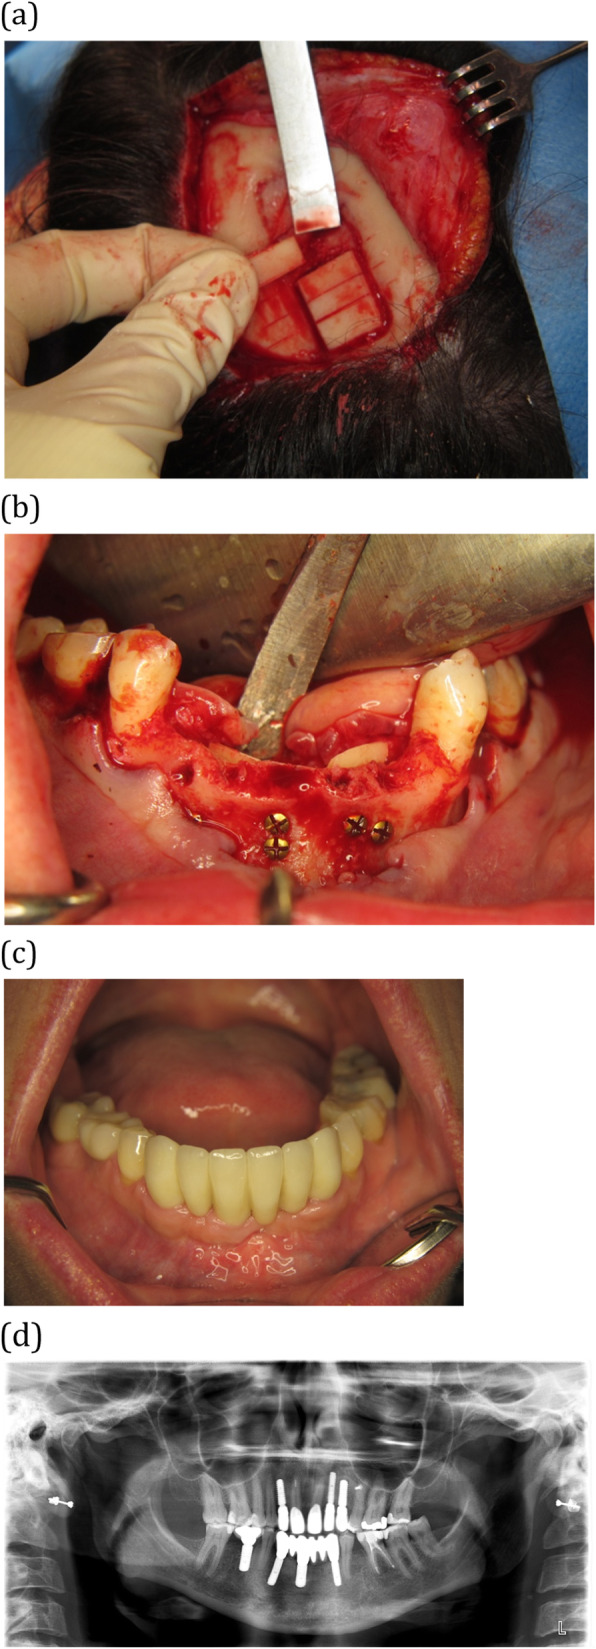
A 43-year-old female with oligodontia with missing tooth numbers 12, 22, 23, 35, 33–43 and 45. a Harvesting of outer table calvarial bone blocks. The blocks were harvested piece-by-piece. b Reconstruction of the mandible at tooth locations 33–43 by placing the calvarial grafts lingually. The grafts were fixed with screws from the buccal side. The implants were placed after 4 months. c Clinical image of the end result. A fixed bridge was made in the region 33–43. d Orthopantomogram of the end result at 21 months follow-up showing negligible marginal bone loss around the implants
